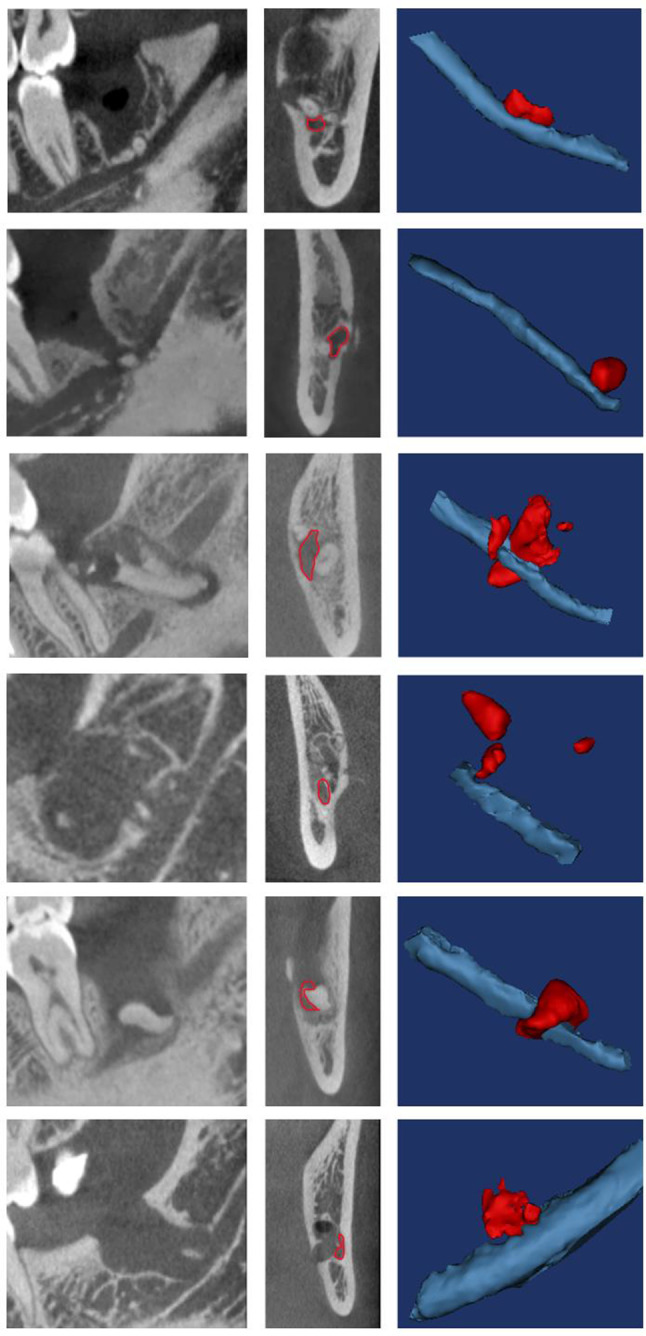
CBCT showing the positional relationship between fractured roots and the mandibular canal (red outline). Mimics software was used to reconstruct the 3D relationship between fractured roots (red) and the IAN (blue)
